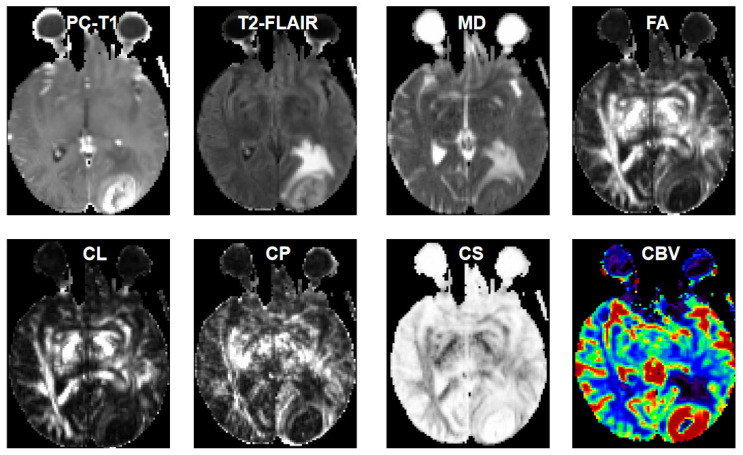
A 51 year old male patient with metastatic lung adenocarcinoma in the left occipital lobe. Axial post contrast T1-weighted, and T2-FLAIR images show ring-enhancement and extensive edema. DTI derived maps (MD, FA, CL, CP and CS) are shown and CBV map showing high perfusion from CER of neoplasm. Please note lower CBV value of this BM from IPR than that of GBM (shown in Figure 4).
